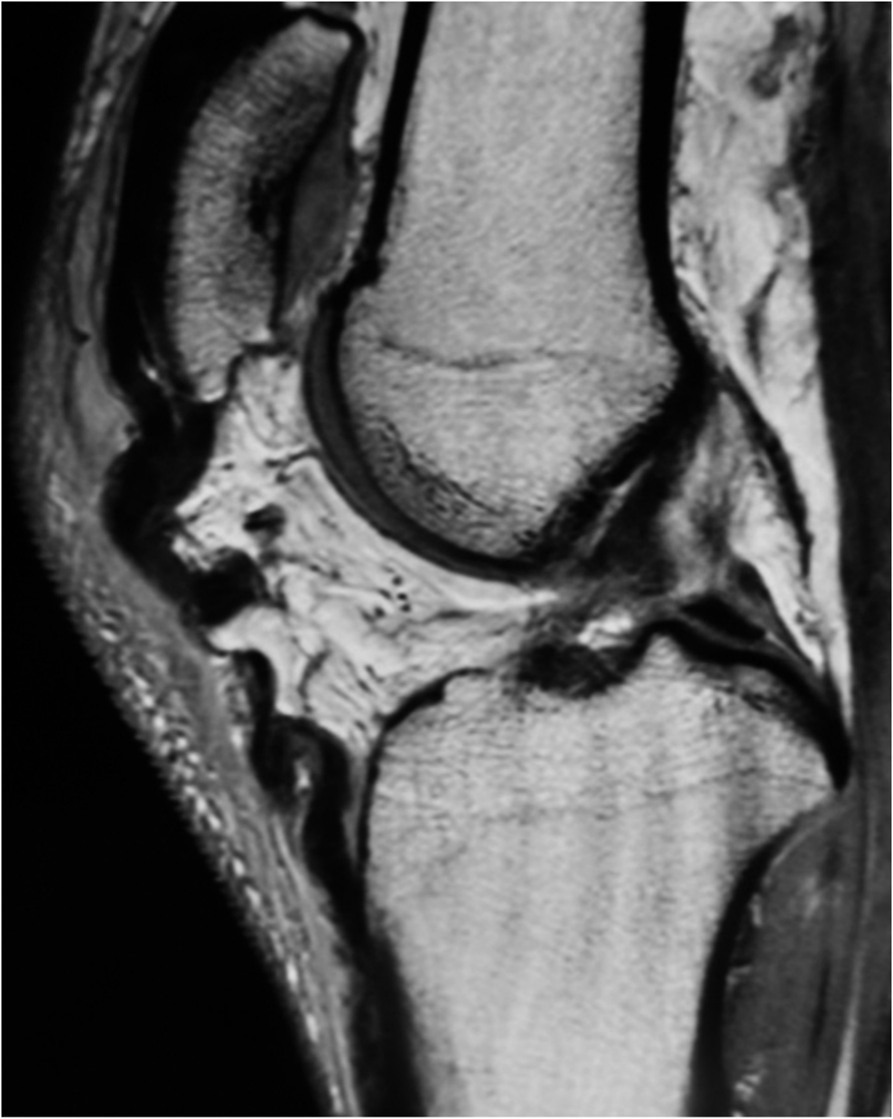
Proton magnetic resonance image (sagittal view) of case 2 demonstrating hemorrhage and focal discontinuity, consistent with a rupture of the left patellar tendon.
